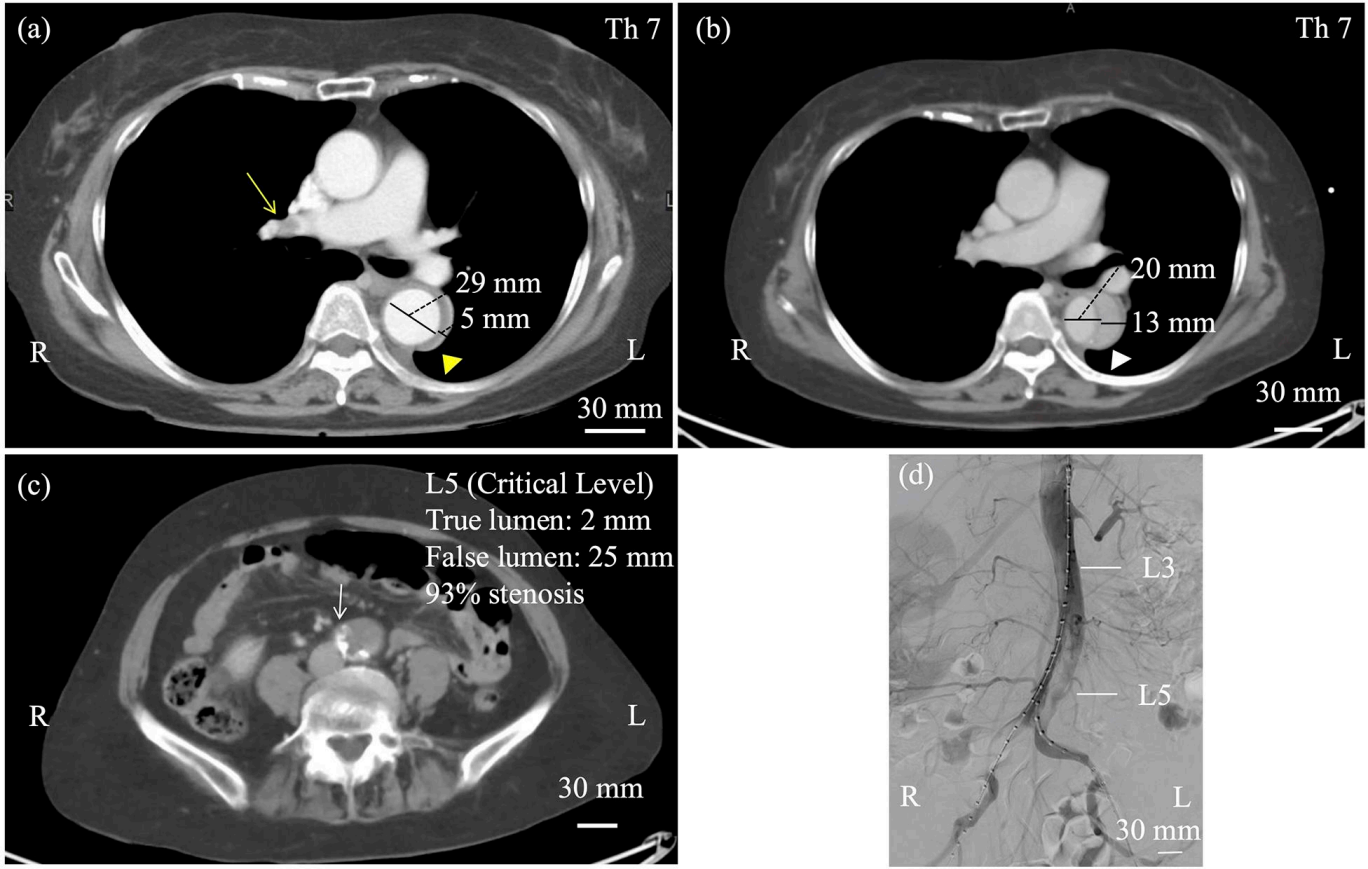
(a) Contrast-enhanced computed tomography at day 6 showed right pulmonary artery embolism (yellow arrow) and a thrombosed false lumen in the descending aorta (yellow triangle). The true lumen measured 29 mm, and the false lumen 5 mm at this time. (b) At day 12, computed tomographic angiography (CTA) revealed an open false lumen (white triangle) and narrowing of the true lumen (c, white arrow), with reduced flow on angiography. At the Th7 level, the true lumen measured 20 mm and the false lumen 13 mm, whereas at the critical L5 level, the true lumen was narrowed to 2 mm and the false lumen expanded to 25 mm. Objective perfusion parameters such as ankle–brachial index (ABI) and renal function were referenced for classification, as shown in the Results section (d).
